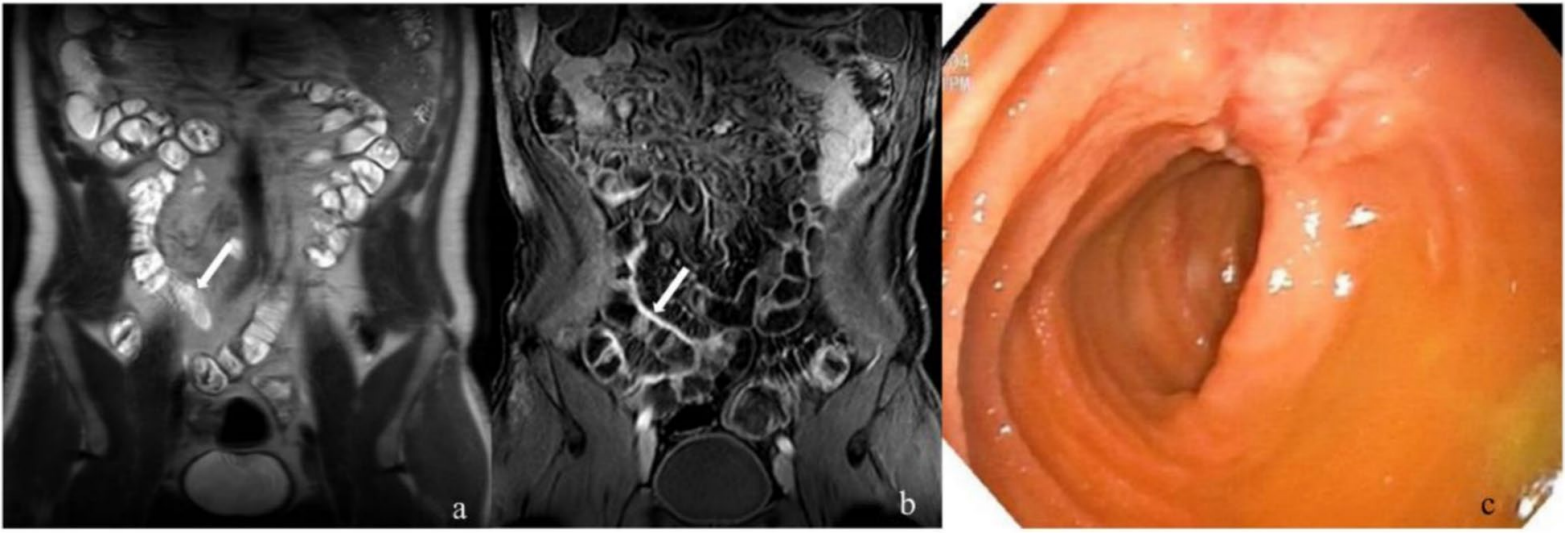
A 29-year-old man with Crohn’s disease. a Coronal T2-weighted imaging before endoscopy: *arrow* points the lesion in the terminal ileum with no significant thickening of the intestinal wall and mild hyperintensity. b Coronal enhanced T1-weighted imaging with moderate and homogenous enhancement (*white arrow*). c The endoscopy could pass
